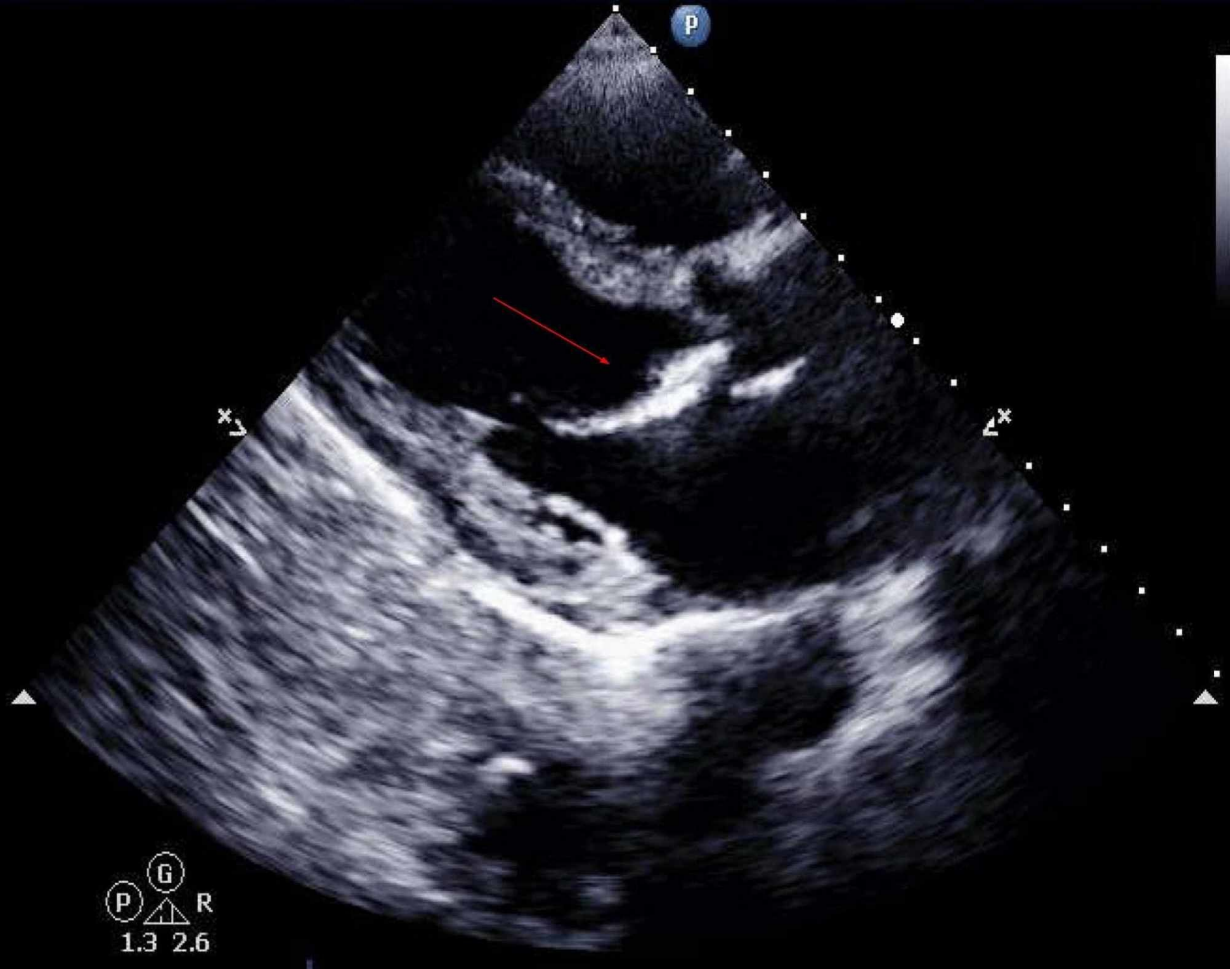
Transthoracic echocardiogram (long axis view) showing calcified anterior mitral leaflet The red arrow showing calcified anterior mitral valve leaflet
